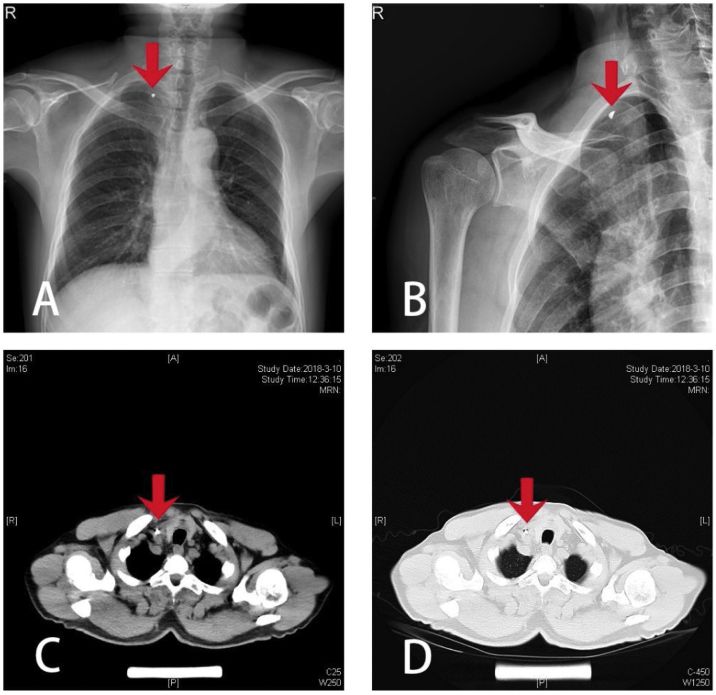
Preoperative imaging: (A) frontal chest radiograph; (B) tangential clavicular joint radiograph; (C) chest CT plain scan (mediastinal window); (D) chest CT plain scan (pulmonary window); (red arrow pointing at the foreign object).
